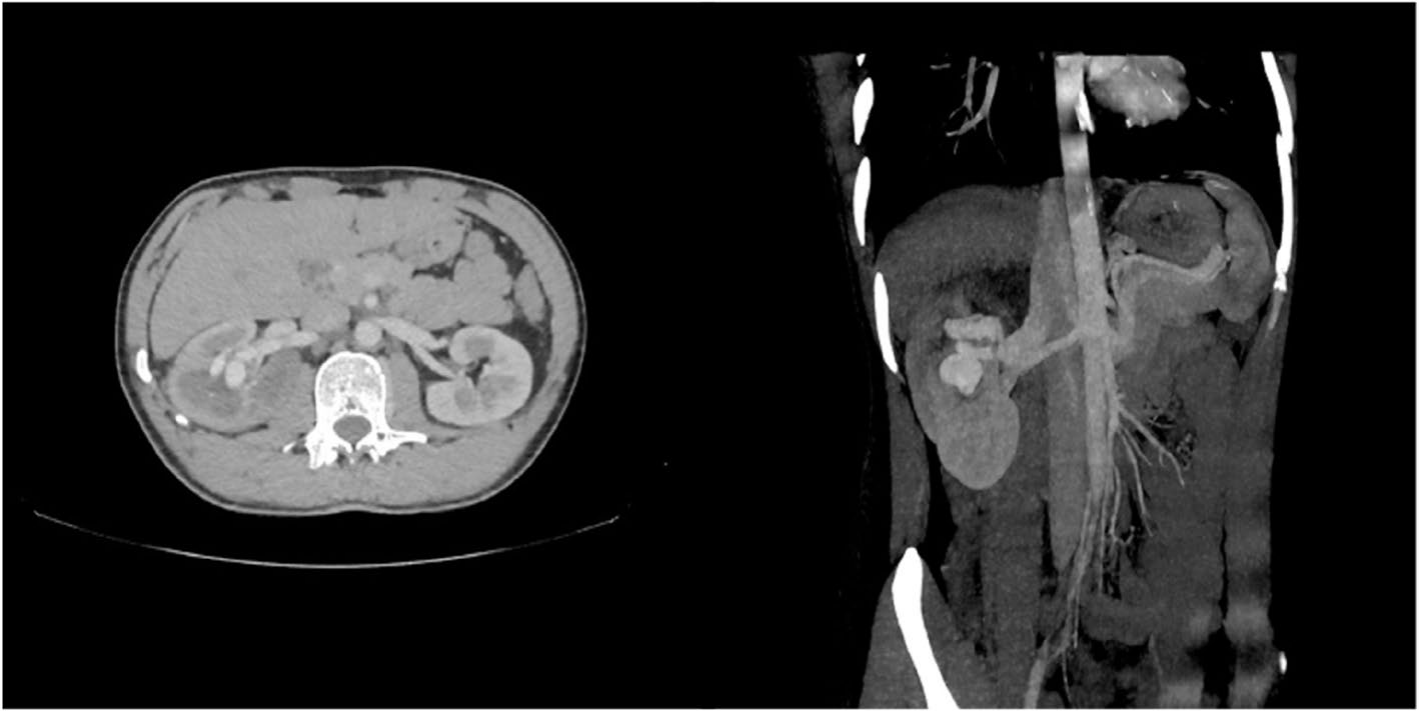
Contrast enhanced computed tomography axial and coronal plane with MIP reconstruction demonstrating a right RAVF
